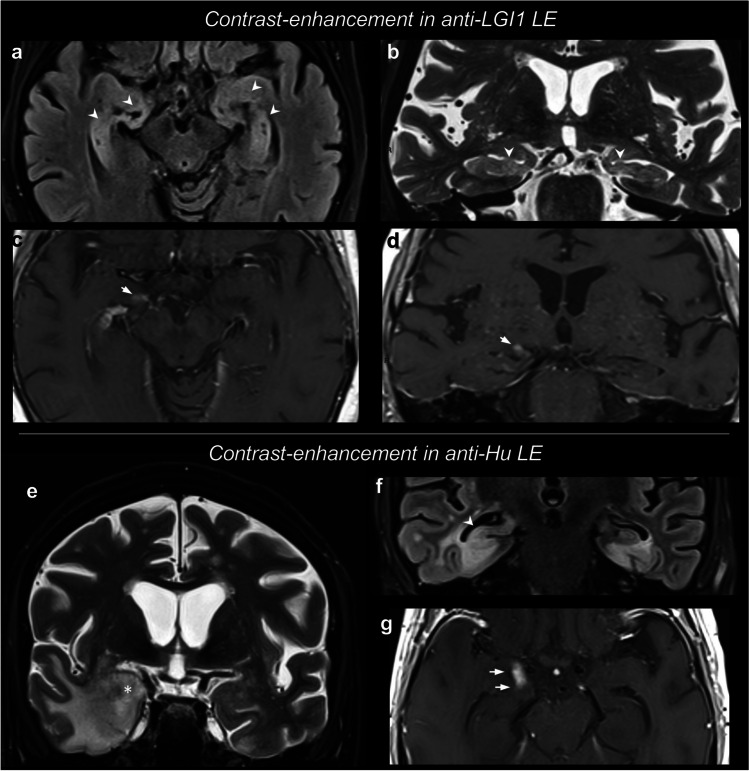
Limbic encephalitis with contrast-enhancement. Both cases presented with an encephalitic bilateral involvement of the medial temporal lobe (MTL) structures, and unilateral areas of contrast-enhancement in the right amygdala (arrows). The arrowheads show the bilateral areas of T2/FLAIR hyperintensity in the MTL. The anti-LGI1 AE case shows more symmetric involvement of the amygdalae and hippocampi on T2/FLAIR. The anti-Hu AE case shows an asymmetric pattern, with a more predominant swelling and hyperintensity of the right amygdala (asterisk in e) and hyperintensity of the right body of the hippocampus (arrowheads in f). These alterations are associated with MTL grey and white matter hyperintensity, including edema with finger-like appearance
